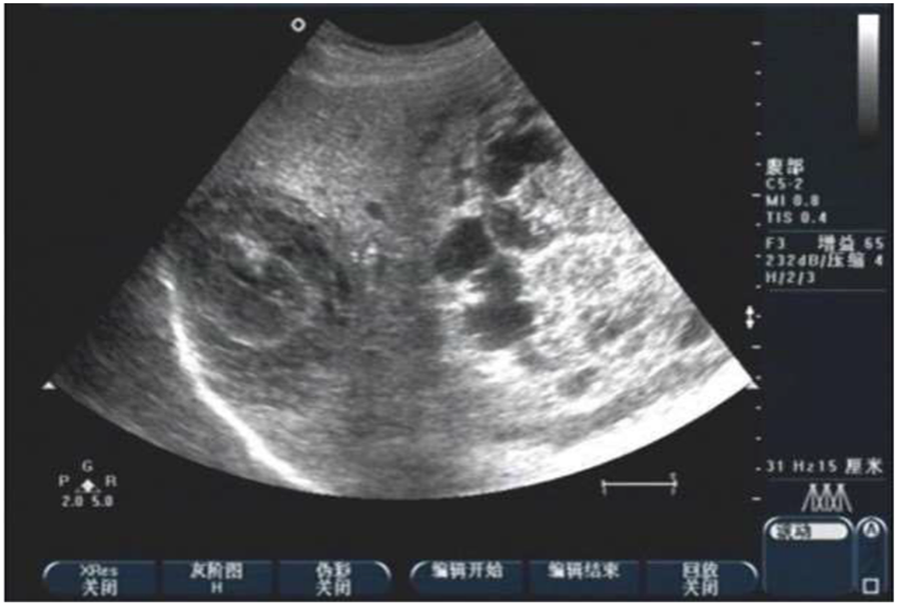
Ultrasound images of the mass in the right liver and abdominal cavity.
